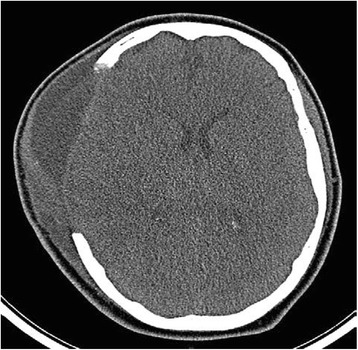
Computed tomography (axial) showing a large right-sided craniectomy and expansion of brain parenchyma away from the midline
